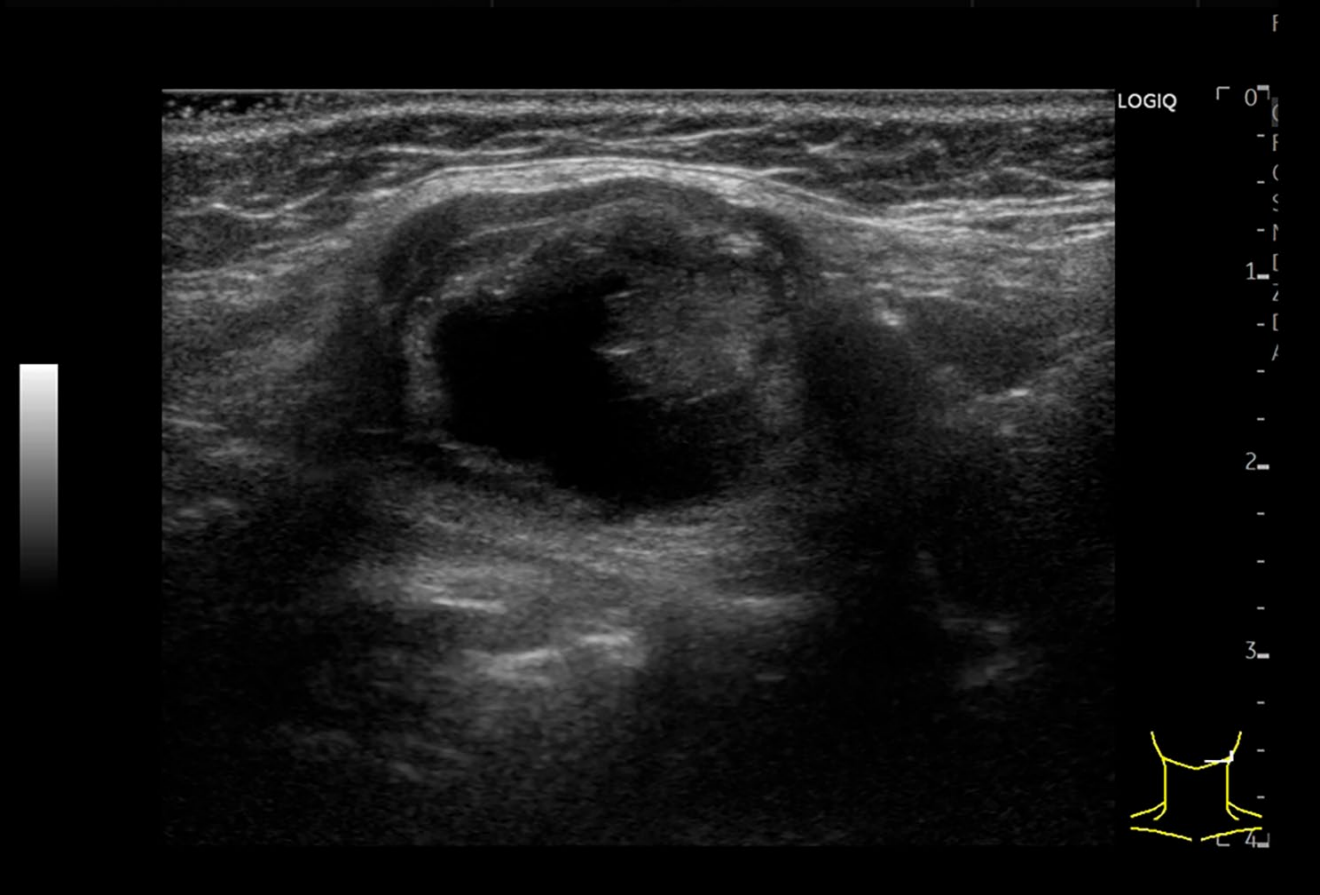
Ultrasonographic images of the tumor. The tumor was located in the left submental region and had a low internal density with mixed papillary hyperechogenicity at the internal margin
